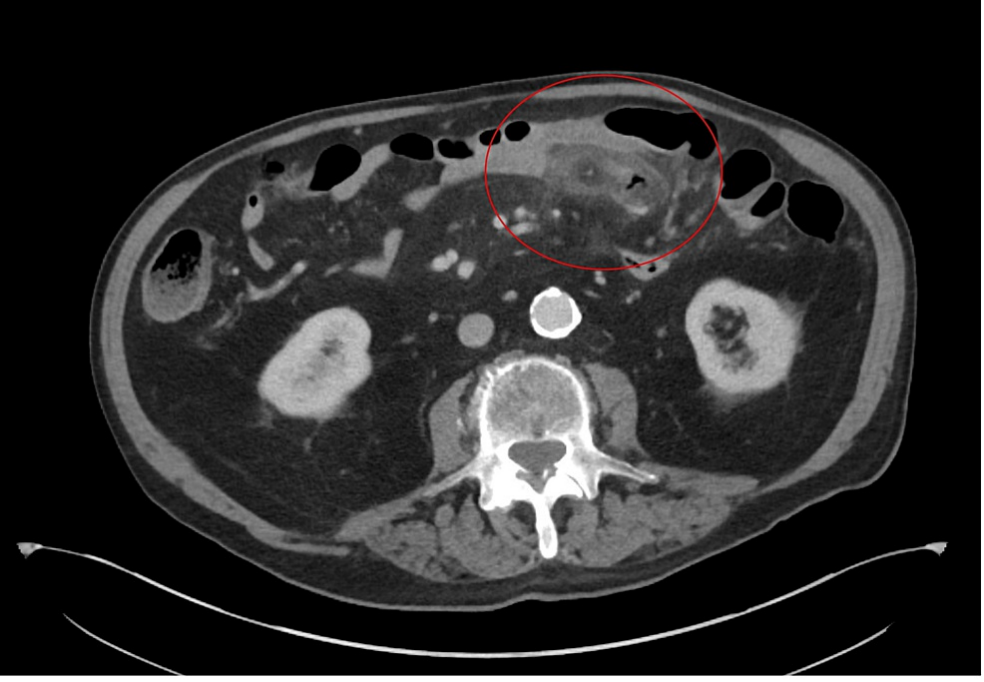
Axial CT image. In the left side of the abdomen, there is a perforation (red circle), which appears to involve a small bowel with extraluminal air and an intense inflammatory reaction.
